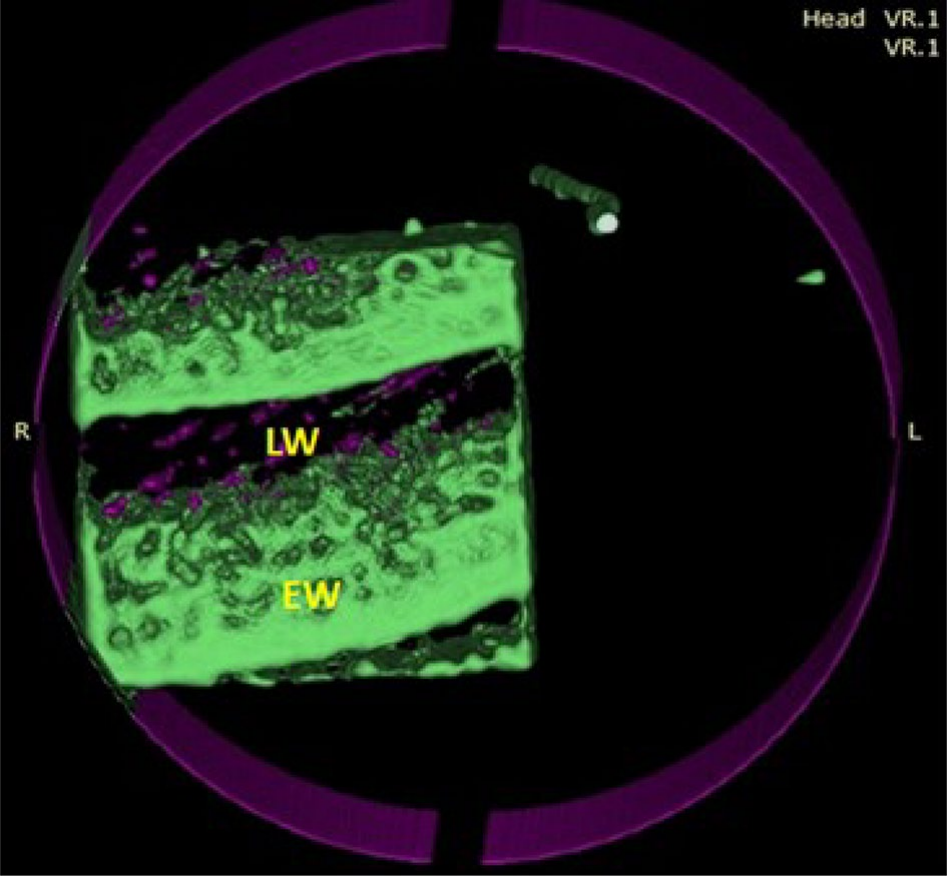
CT scanning of teak wood infected by *P. chrysogenum* after 36 months of the artificial inoculation. *LW* latewood, *EW* early wood.
